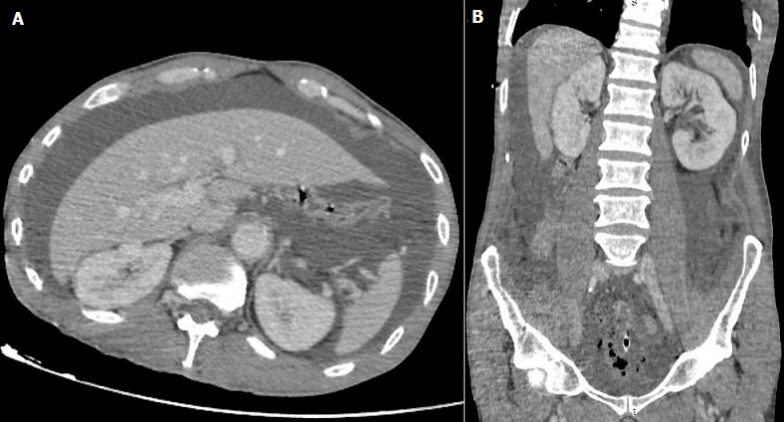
A) axial view on CT scan showing large amounts of intraperitoneal fluid; B) coronal reconstruction of the CT scan showing large amounts of ascites, associated with free intraperitoneal air in the pelvis
